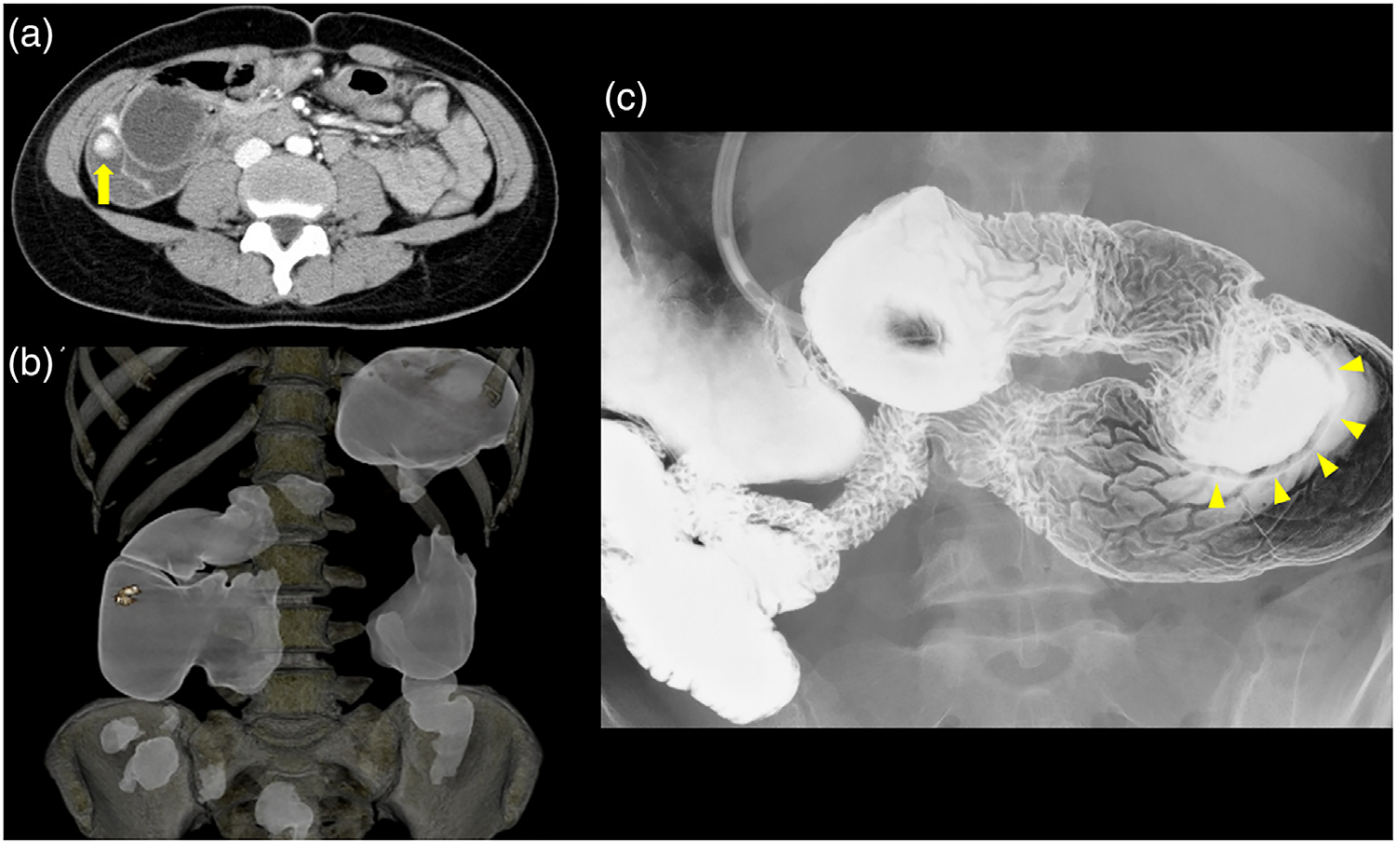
Radiographic examinations conducted for tarry stool and anemia associated with abdominal distension and regurgitation. (a) An abdominal computed tomography (CT) slice revealing dilatation of the duodenal bulb, and a membranous structure in the second part of the duodenum with contrast media extravasation (yellow arrow). (b) A representative 3D‐CT image showing the typical “double‐bubble sign”. (c) A representative barium duodenography image obtained with the patient in the prone position showing a membranous structure of approximately 3 mm in thickness arising from the second part of the duodenum (yellow arrowhead).
